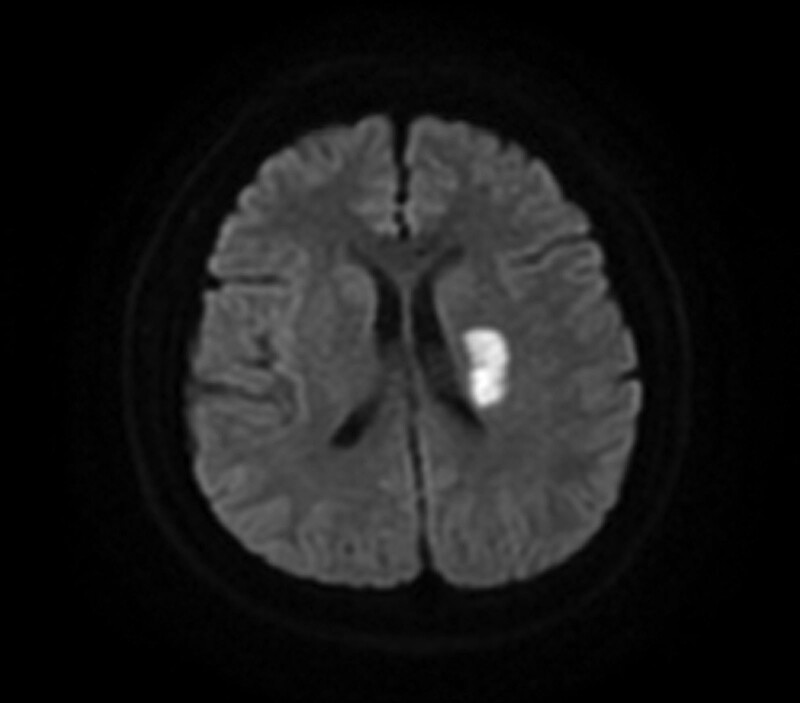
A cranial MRI showed a subacute cerebral infarction in the left lateral ventricle. MRI = magnetic resonance imaging.
